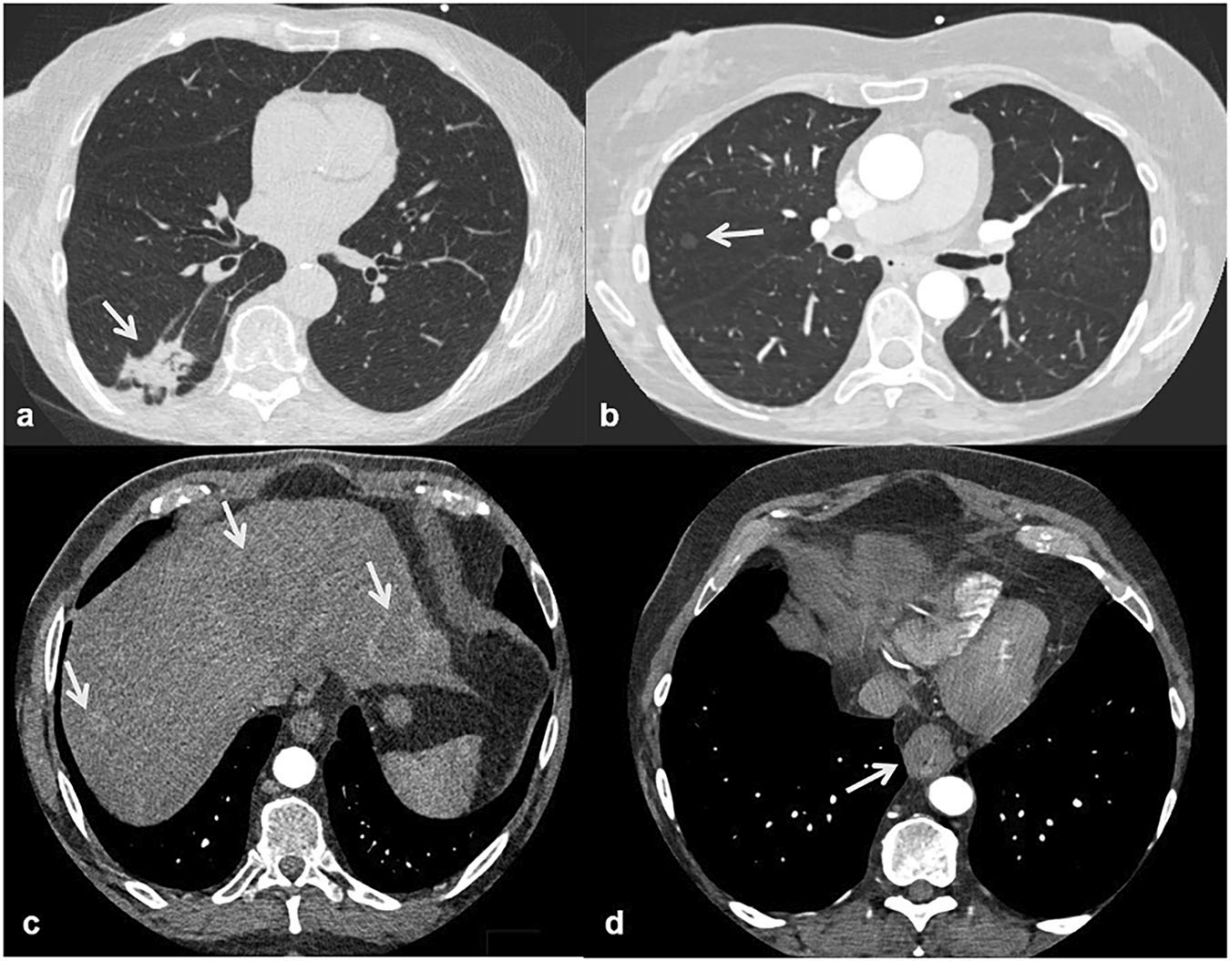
Examples of malignant extra-coronary findings detected on coronary computed tomography angiography (CCTA). a Pulmonary lesion confirmed as non-small cell lung cancer (NSCLC). b Pulmonary ground-glass nodule confirmed as NSCLC (adenocarcinoma subtype). c Liver lesions confirmed as metastases from pancreatic cancer. d Esophageal lesion was confirmed as esophageal adenocarcinoma
